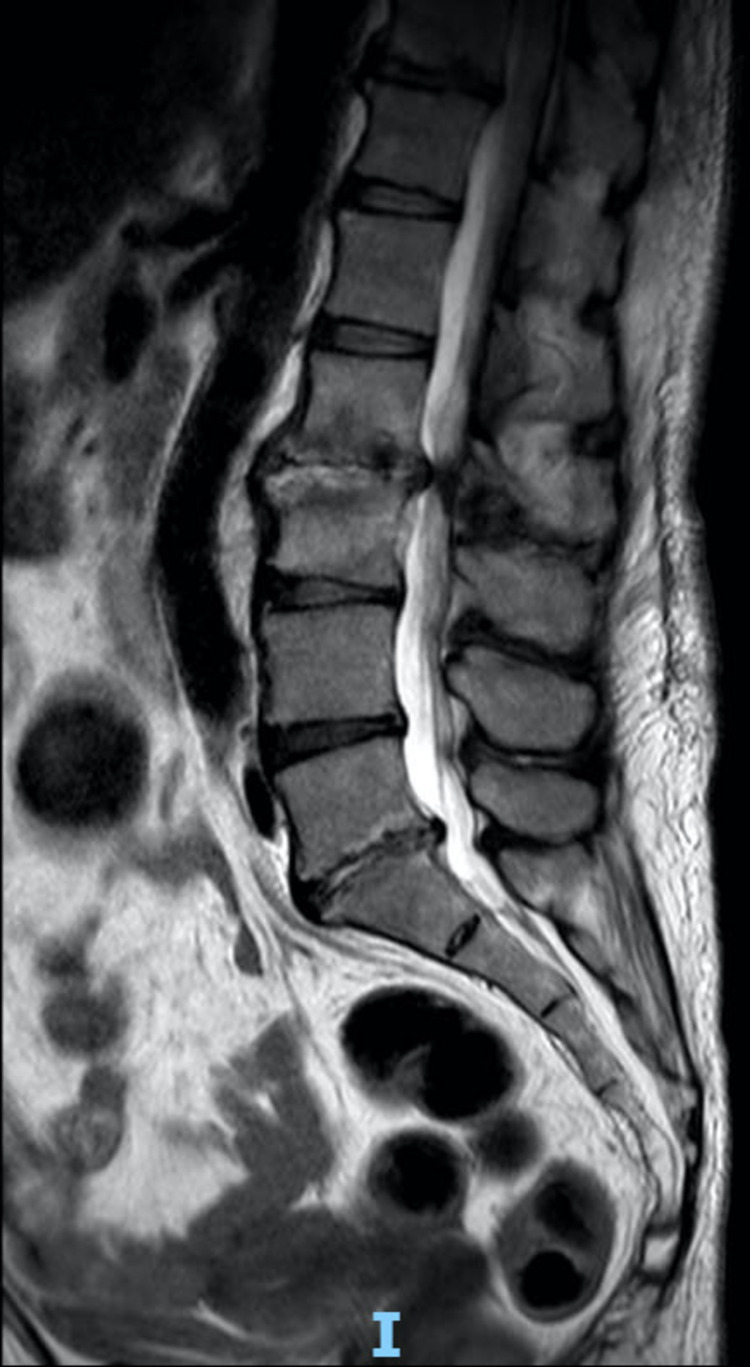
Sagittal MRI view of the spine (T2-weighted) with a hypersignal in the L2L3 and L5S1 disc spaces
